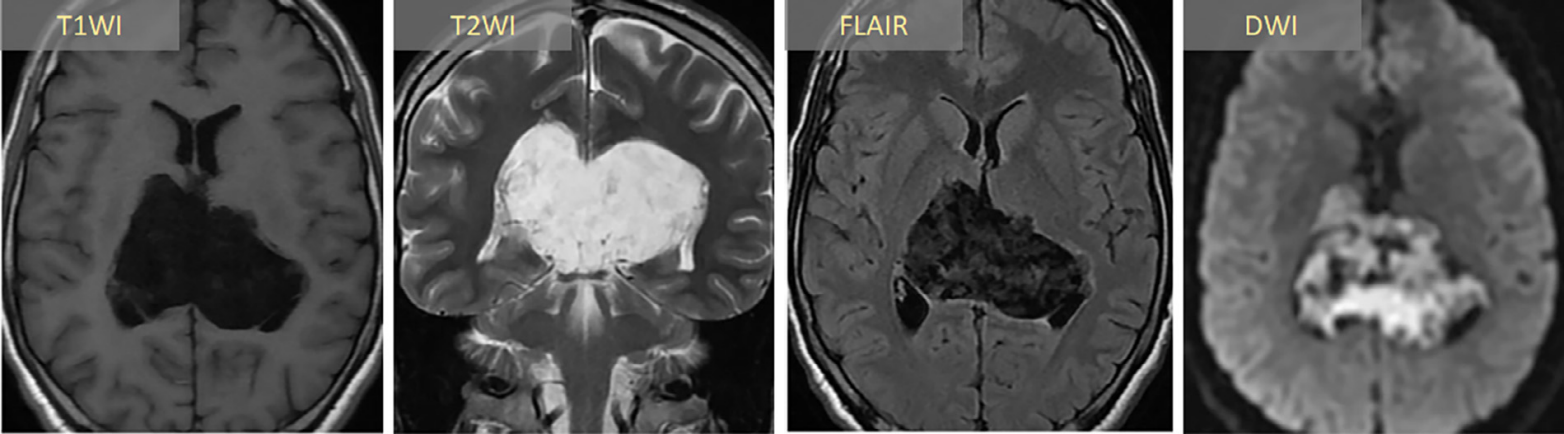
Axial MRI sequences demonstrate a large biventricular epidermoid tumor with posterior extension to the pineal region. The lesion exhibits: (A) heterogeneous hypointensity on T1WI; (B) hyperintensity on T2WI; (C) incomplete signal suppression on FLAIR; and (D) marked diffusion restriction on DWI. *T1WI: T1-weighted imaging; T2WI: T2-weighted imaging; FLAIR: fluid-attenuated inversion recovery; DWI: diffusion-weighted imaging.*
